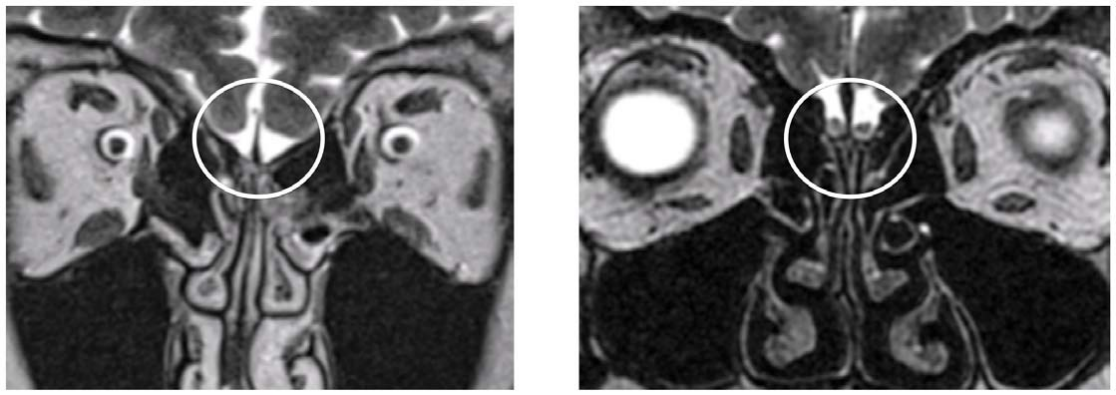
Structural magnetic resonance image of an isolated congenital anosmic patient (left). Within the marked region an olfactory bulb is missing. This becomes obvious compared to the healthy person visualized in the right picture.
